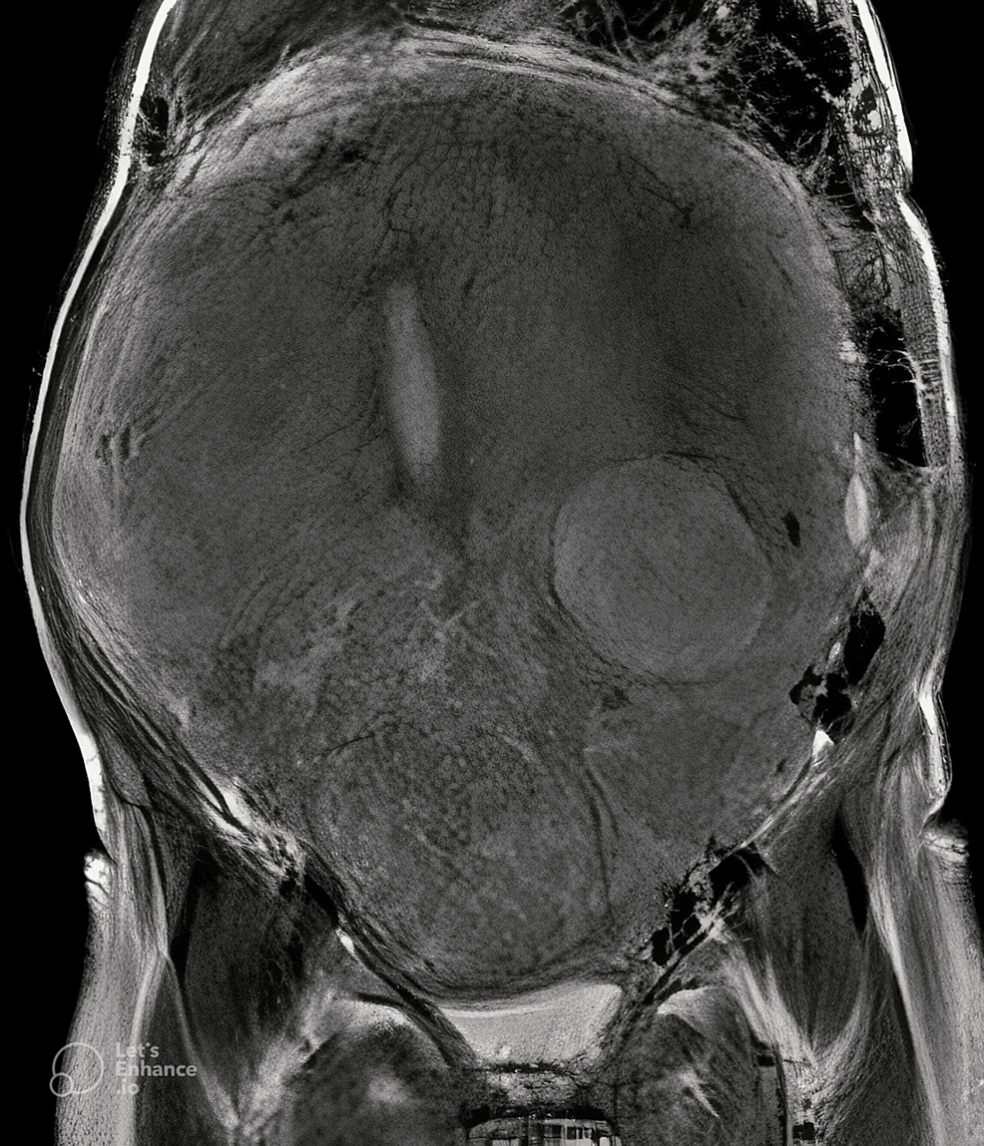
Coronal T2-weighted imaging showing a grossly enlarged uterus with multiple T2 heterogenous signal intensity intramural fibroids in the lower uterine segment and extensively thickened myometrium in the fundal region and upper uterine segment (1.5 Tesla MRI).
